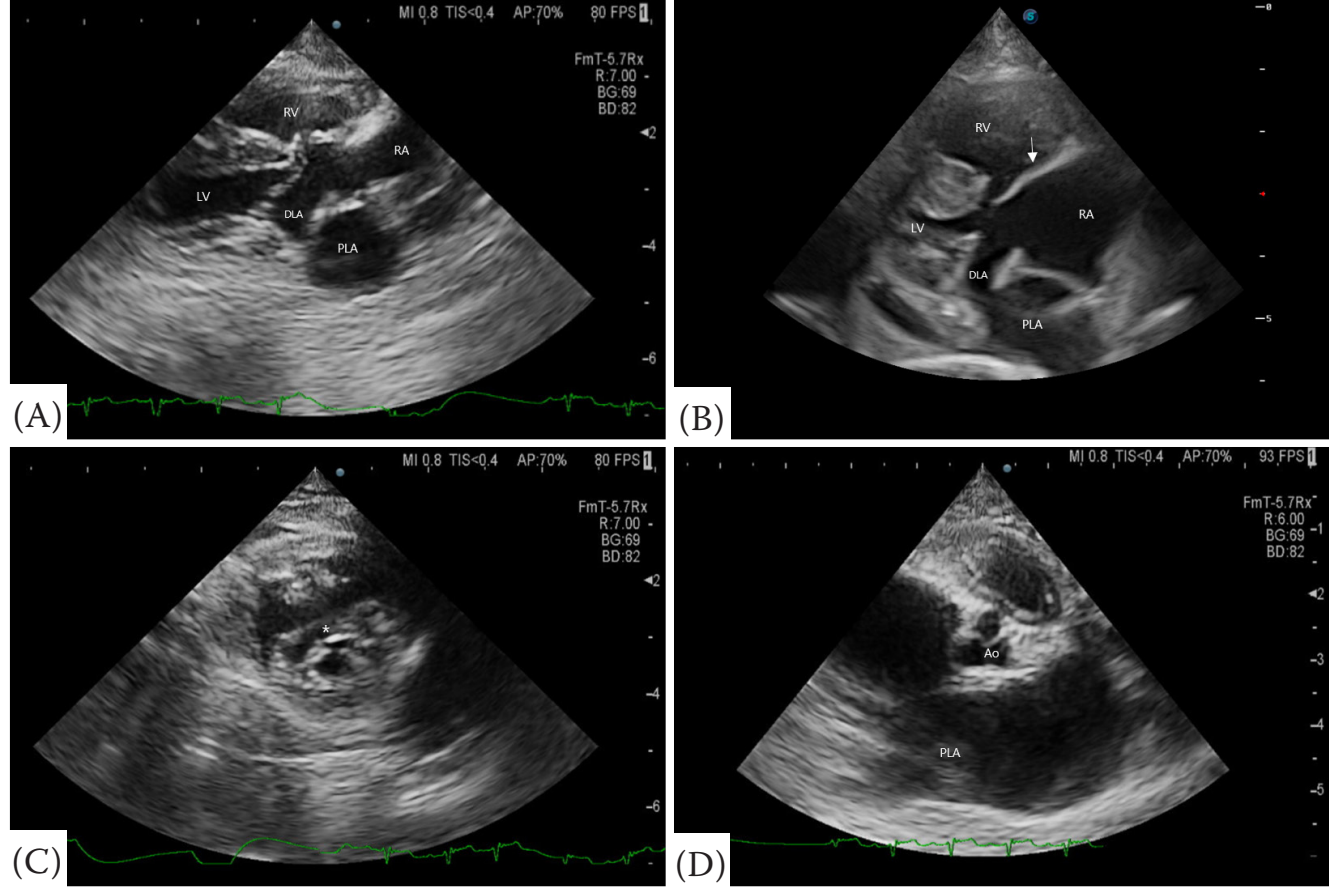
Two-dimensional echocardiography in the right parasternal 4-chamber (A, B) and short-axis views (C, D) (A) The echocardiographic view shows a membrane dividing the left atrium into two chambers, with the proximal chamber enlarged and the distal chamber reduced in size. (B) A large defect is visible in the lower part of the atrial septum, with elongation of the anterior or posterior tricuspid valve (TV) (arrow), although the septal TV is absent. (C) Flattening of the interventricular septum (asterisk). (D) Dilation of the proximal LA
